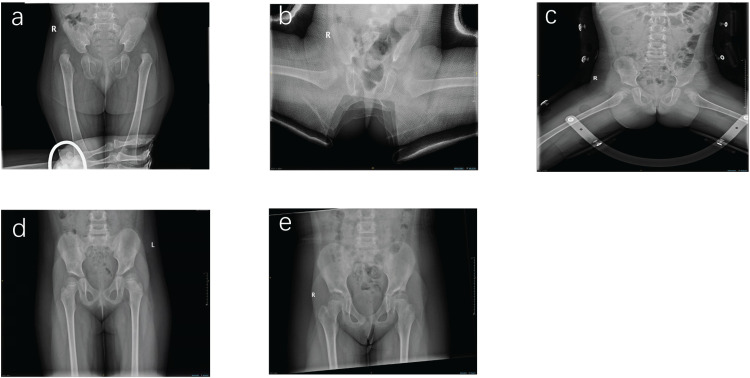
Radiological follow-up of a typical patient. The patient, a 20-month-old girl with bilateral DDH classified as IHDI grade IV. a) Pre-operative X-ray. b) Closed reduction and hip spica cast fixation were performed. c) After the cast was removed, an abduction orthosis was utilized. d) Pelvic radiographs after 24 months of follow-up. e) Pelvic radiographs after 80 months of follow-up.
